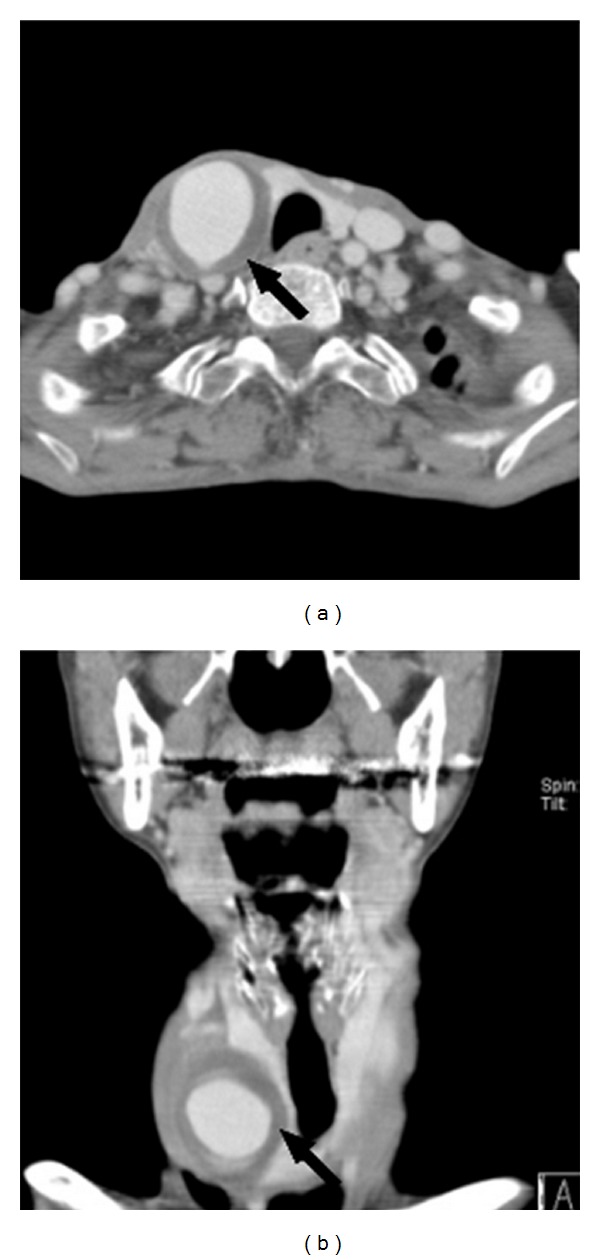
((a) and (b)) Contrast-enhanced CT angiograms demonstrating a 2.5 × 3.4 cm sized thrombosed pseudoaneurysm (arrow) in the right common carotid artery.
